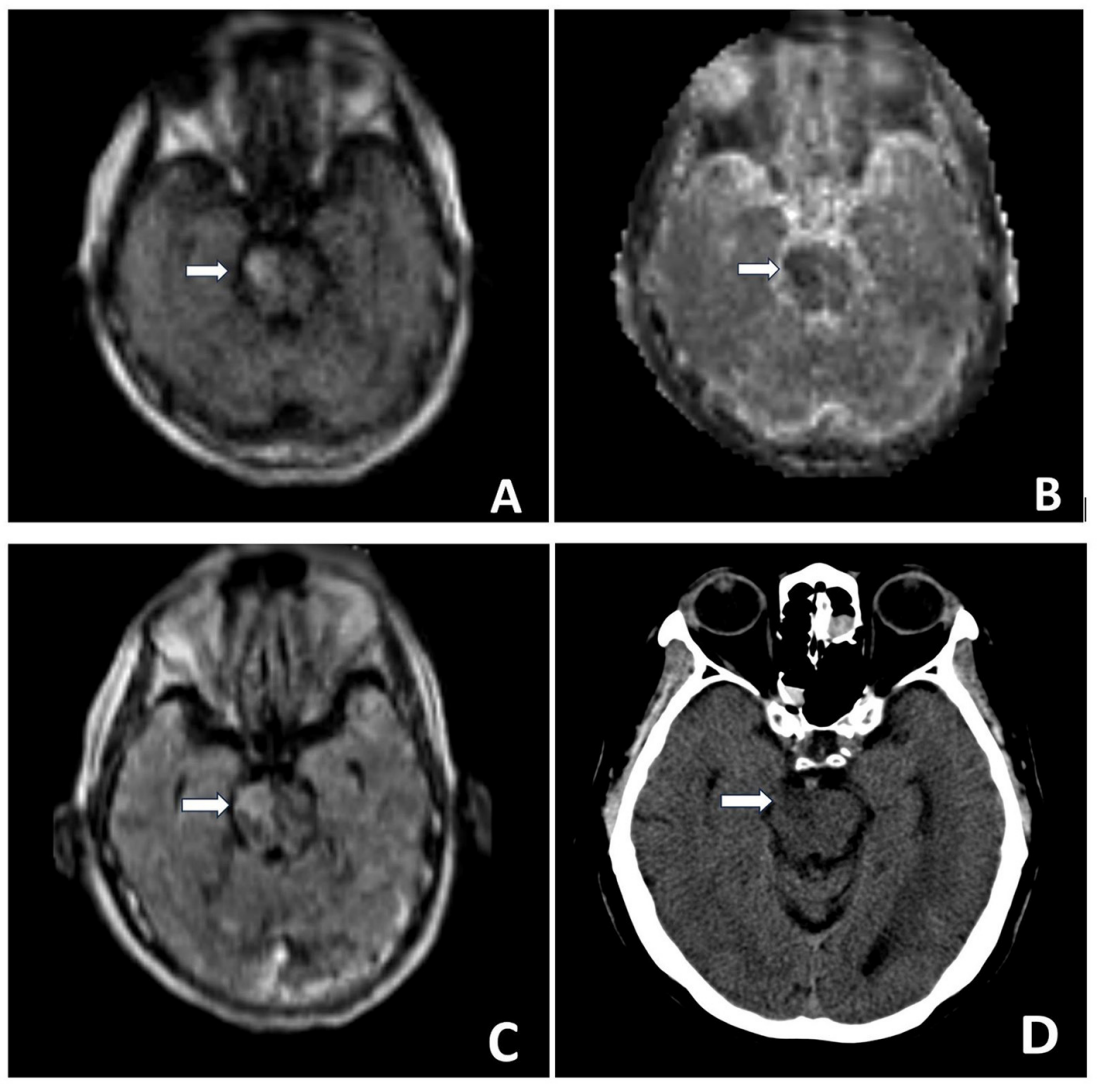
Brain MR POC MRI DWI sequence (A), ADC sequence (B) demonstrate right ventral pontine restricted diffusion. T2/FLAIR sequence (C) demonstrates right ventral pontine hyper-intense signal. Head CT performed the same day (D): Hypo-attenuation at the site of right pontine infarction, but at times difficult to confidently diagnose posterior fossa strokes on CT given artifacts in the area. MRI exam helps verify diagnosis. MR Images were obtained using the Swoop Hyperfine Portable MR Imaging System (64 mT) at the University of California, Irvine Medical Center.
